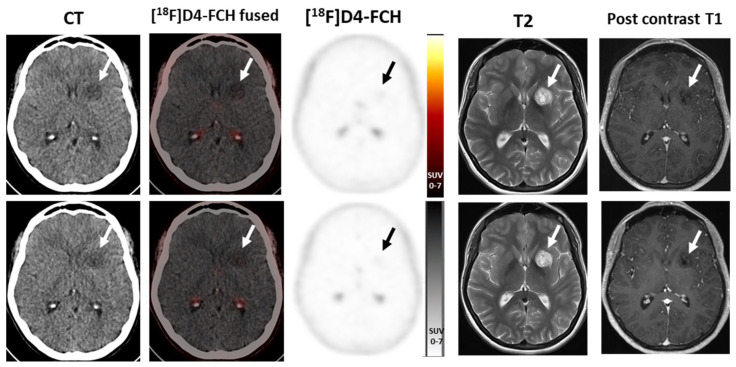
[^18^F]D4–FCH PET/CT in a left thalamic low-grade glioma 6 months apart (top row, baseline; bottom row, 6 months later) showing minimal tracer uptake above background in the lesion, stable between scans, high signal on T2–weighted MRI imaging with no significant enhancement post-contrast. (Note physiological activity in the choroid plexus). Stable on continued follow-up 6 years later (not shown).
